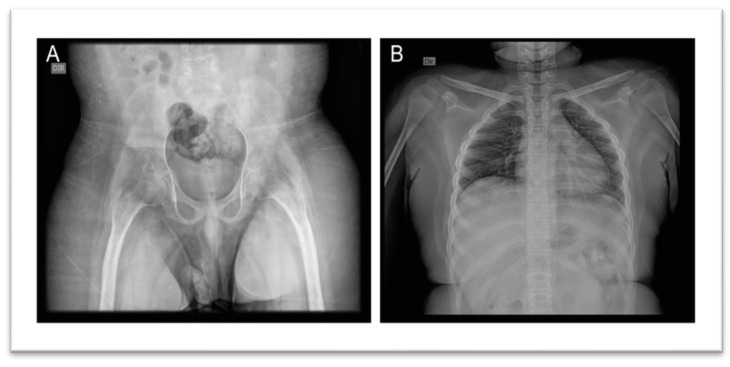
Radiographic Images. (A) Pelvis: Morpho structural changes in both hips characterized by verticalization and flattening of the acetabula, as well as cortical thickening of the femoral diaphysis bilaterally and apparent hypotrophy of the quadriceps muscles. (B) Spine: Slight deviation of the thoracolumbar spine to the right, with no vertebral rotation.
